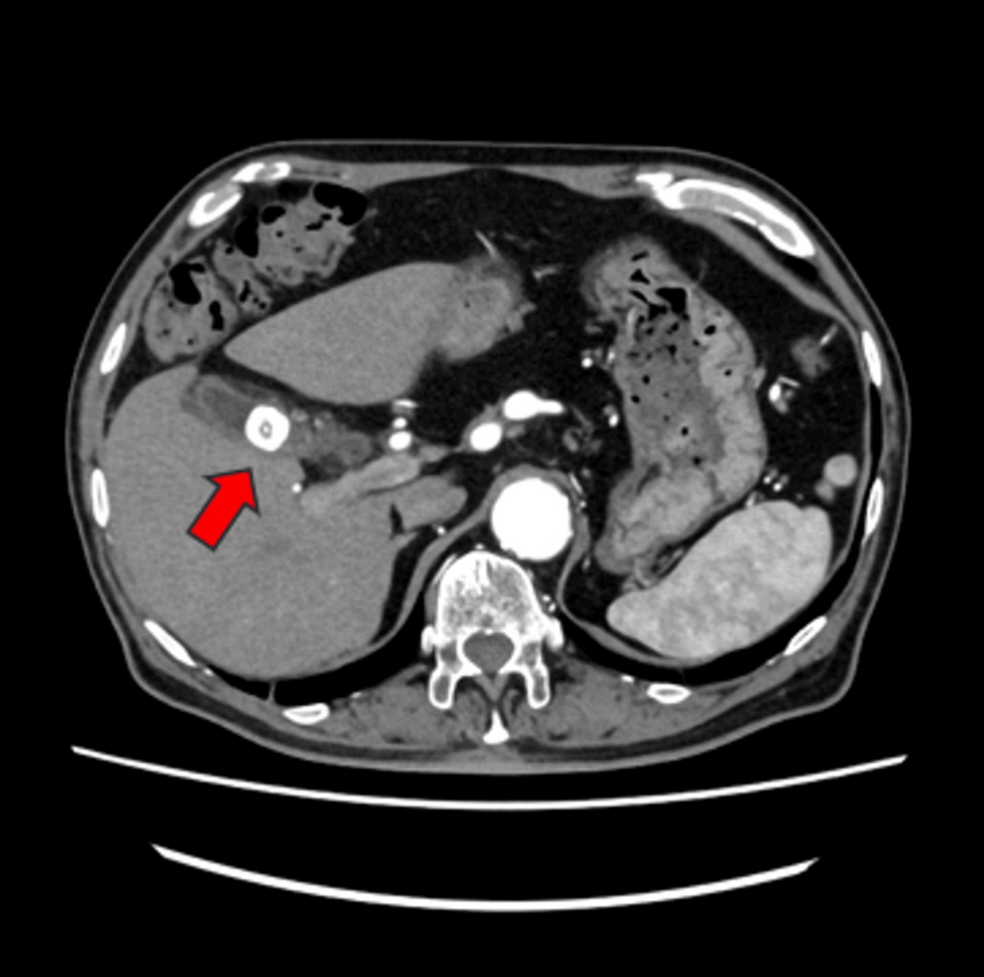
One month after discharge, contrast-enhanced CT revealed improvement in gallbladder swelling (arrow).
